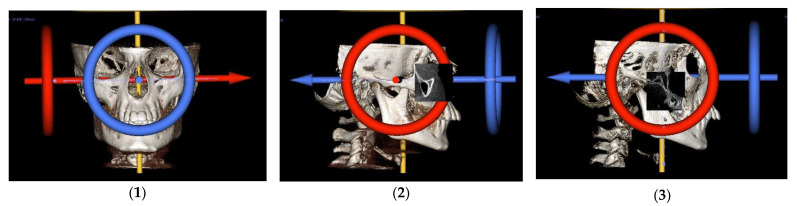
Bilaterally oriented 3D reconstructed CBCT image: (1) frontal view showing grid passing through right and left orbitale in transverse plane; (2) lateral view showing grid passing through right porion and right orbitale in transverse plan; and (3) lateral view showing grid passing through right and left Pterygomaxillary fissures in coronal plane.
